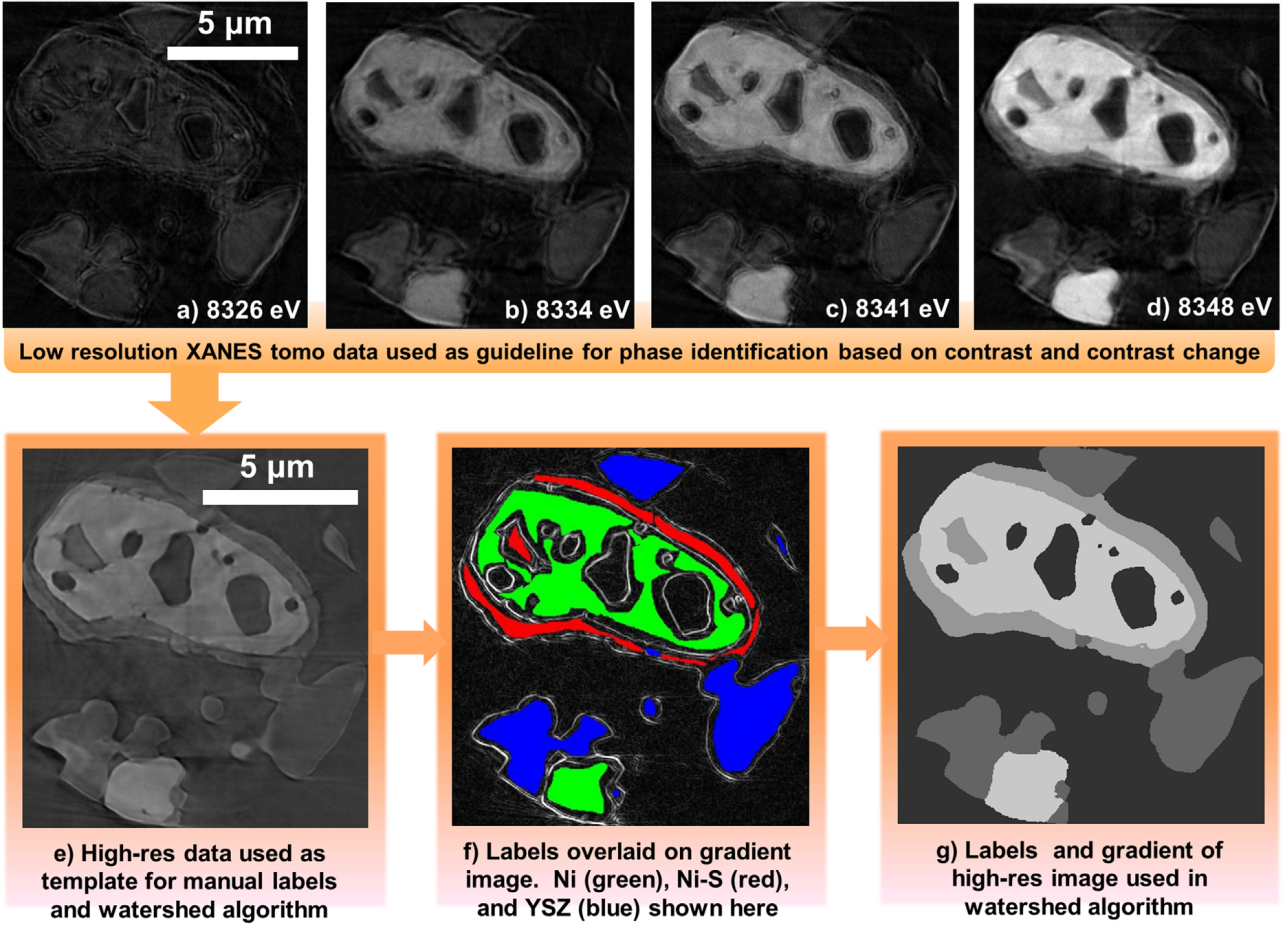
X-ray nanotomography image processing. In (a) – (d) Multi-energy tomography is performed by imaging the sample at four energy levels across the Ni k-edge. Contrast change indicates the presence of Ni, and therefore can identify Ni and Ni-S phases. In (e) a high-resolution tomography at 8350 eV with improved optics provides clear definition of edges and particle boundaries. In (f), phases were labeled based on contrast change and overlaid on the gradient of the high-resolution data. The labels were used as seed regions in a watershed algorithm, creating a segmented structure (g).
